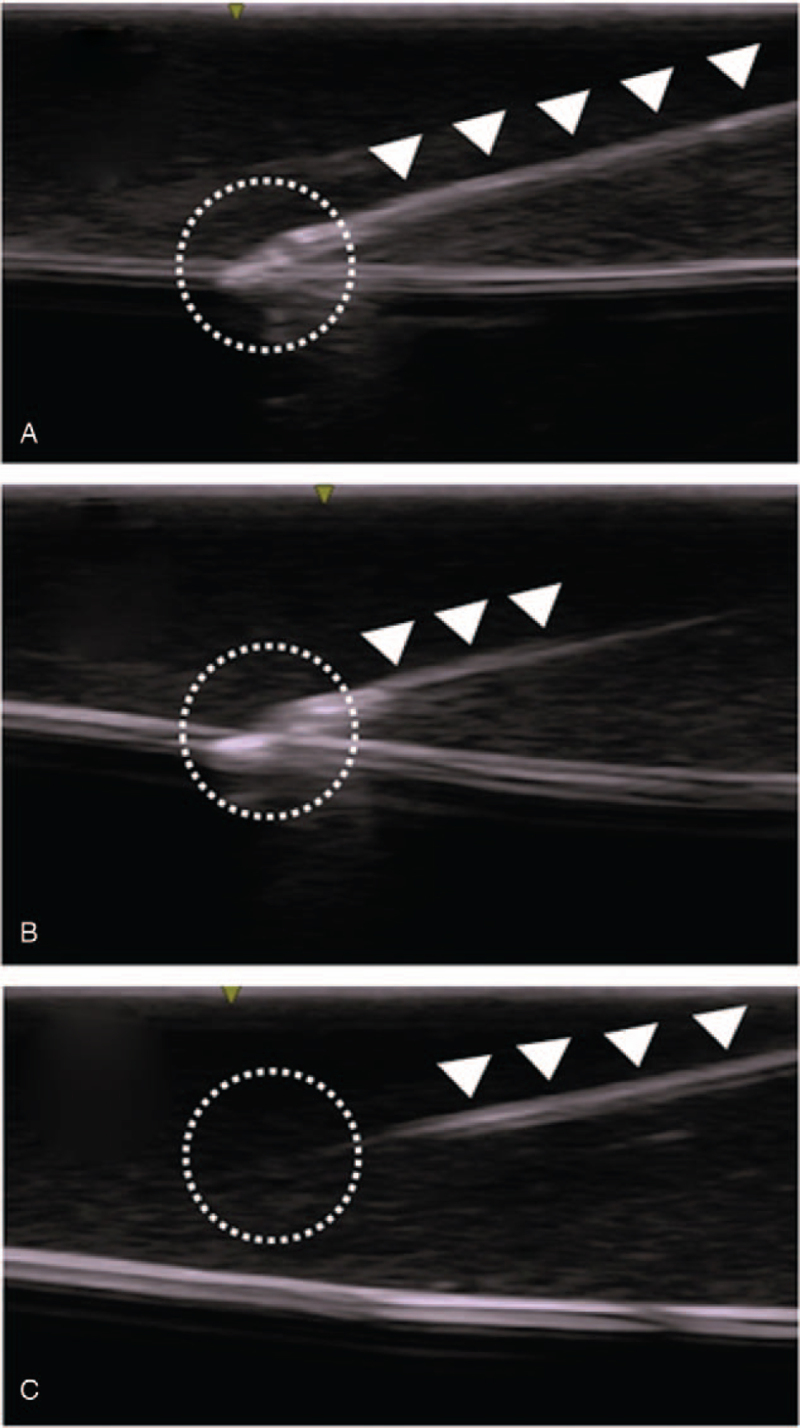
Needle tip visualization-representative images. Ultrasound experts observed the recorded ultrasound views and evaluated whether the needle tip could be classified as “identified” (clearly visible, or visible) or not (“unidentified”). Typical images judged as clearly visible, visible, and unidentified are shown. Panel a: Clearly visible: Needle tip and shaft are seen in full length. White closed triangles indicate the needle. Panel b: Visible: Needle tip and shaft are seen partially. Panel c: Unidentified: Needle tip cannot be observed. The shaft can be seen partially.
